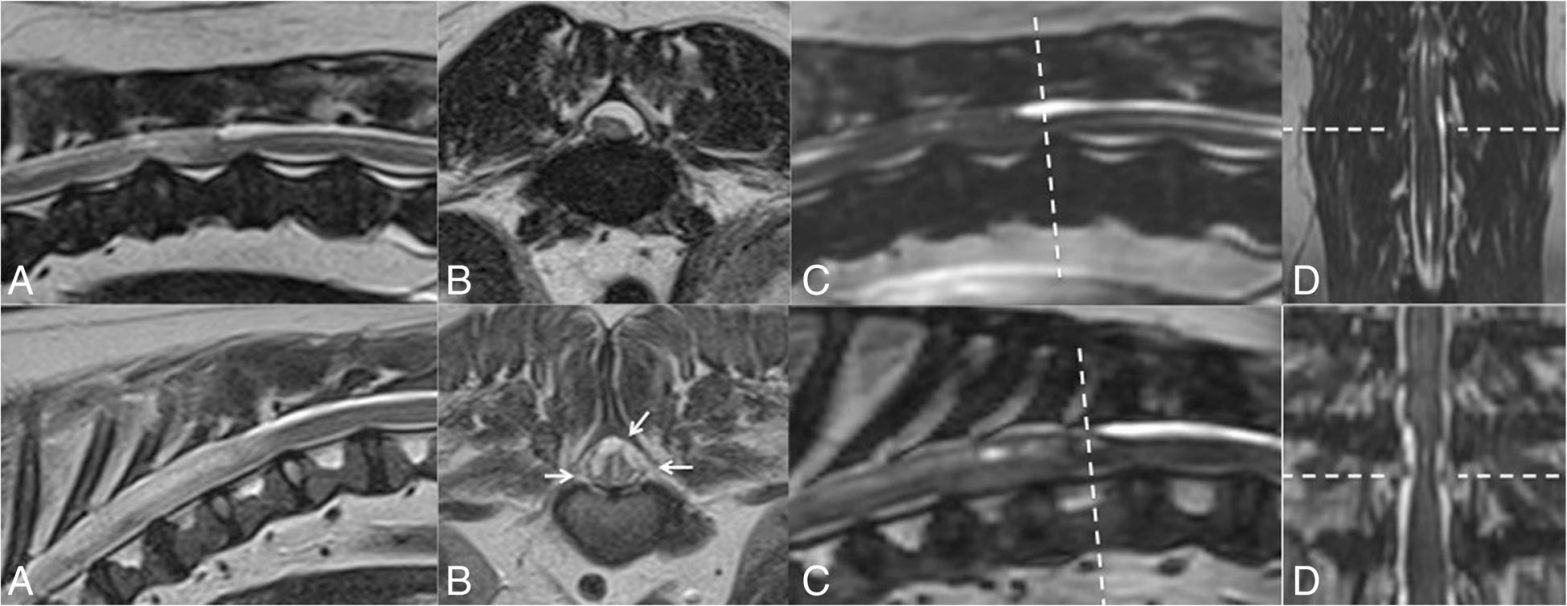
Magnetic resonance imaging of the thoracolumbar spine of case no. 8 (top row) and 14 (bottom row) who display intra-dural spinal cord compression. From left to right; a = T2-weighted sagittal MRI, b = T2-weighted transverse MRI, c = CISS sequence reconstructed in sagittal plane, d = CISS sequence reconstructed in dorsal plane. In case 8, intra-dural spinal cord compression appears to be associated with an expansion of the dorsal arachnoid space, consistent with spinal arachnoid diverticula. CISS imaging highlights this CSF accumulation without apparent attachment of the dura to the pia (dashed line). In case 14, in a transverse plane (lower row image b) the spinal cord appears to develop a ‘stellate’ appearance with multiple hypo-intense bands that cross the arachnoid space (highlighted with short arrows on image b). At the corresponding level on CISS imaging (lower row, images c and d, dashed line) the hypo-intense bands appear lateral to the compressed spinal cord (level marked with dashed line) with an appearance reminiscent of the original description of ‘constrictive myelopathy’ [4]
